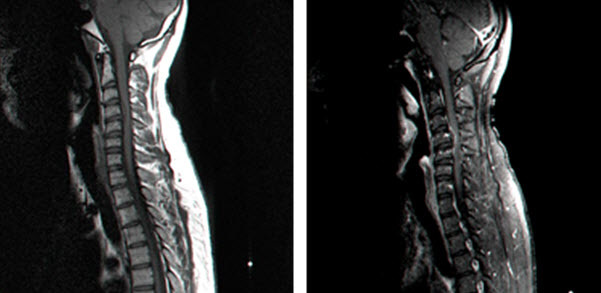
MRI Imaging after Surgery. Left: T1-sagittal epidural hematoma at C3-4 level; Right: T1 STIF sagittal epidural hematoma at C3-4 level.
